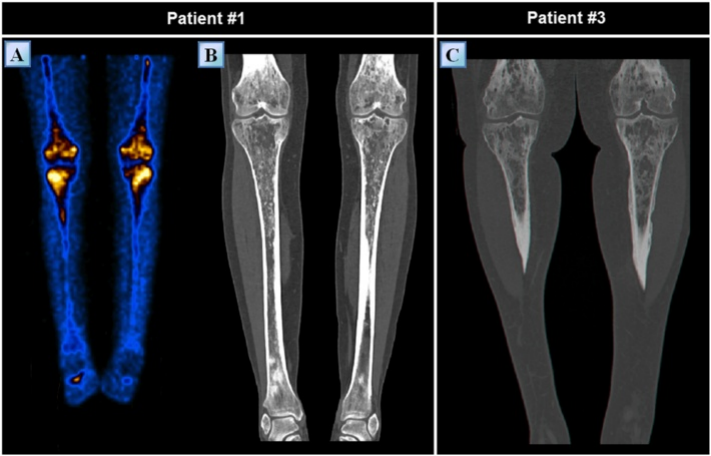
PET and CT findings of the lower limbs of patients #1 and #3 (A) Positron emission tomography showing symmetric, bilateral, abnormally increased intra-medullary uptake of fluorodeoxyglucose in the femurs and tibiae of patient #1 approximately 4.5 years following diagnosis. (B) Computed tomography of the femurs and tibiae of patient #1 at the time of diagnosis, exhibiting periostitis as well as diffuse, irregular intra-medullary sclerosis. These bones are riddled with mixed patchy lesions of sclerotic and lytic nature encased in a markedly thickened cortex. (C) Similar findings can be observed in the computed tomography of patient #3.
